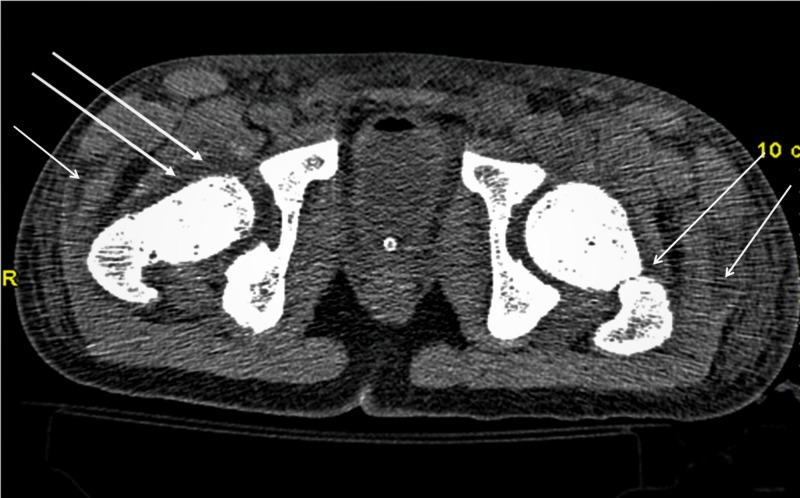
Computed tomography (CT) scan in patient No. 2 with pyomyositis located in both hip areas.
